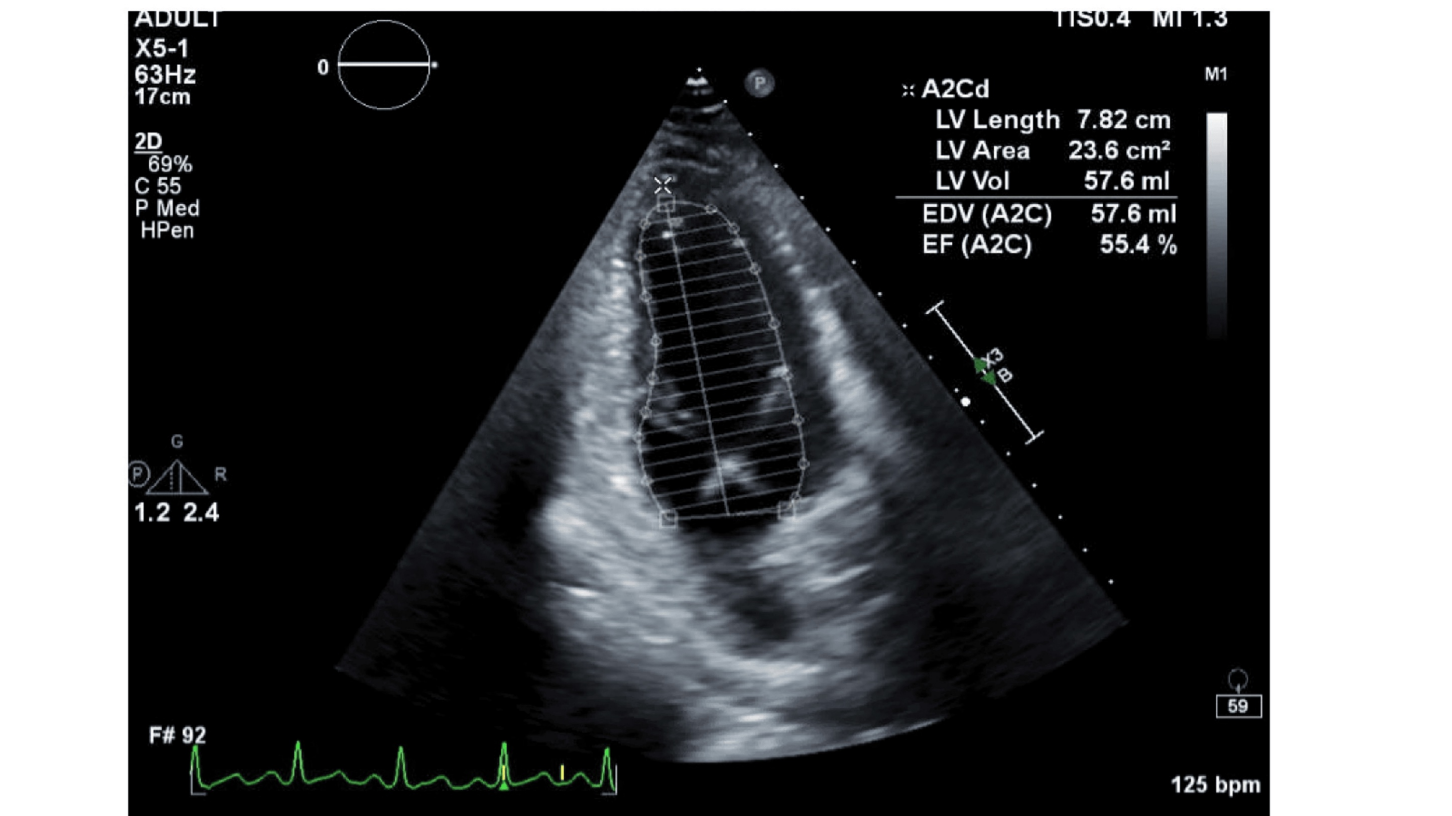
Follow-up transthoracic echocardiogram showing normal left ventricular wall motion and ejection fraction 55% to 60%.
